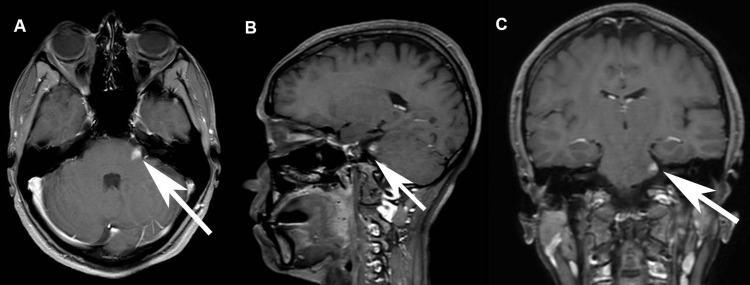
Avid enhancement of small lesion at the origin of the left fifth cranial nerve being partly extra-axial and partly intra-axial in the pons. A: T1W axial MRI brain with gadolinium enhancement B: T1W sagittal MRI brain with gadolinium enhancement C: T1W coronal MRI brain with gadolinium enhancement
